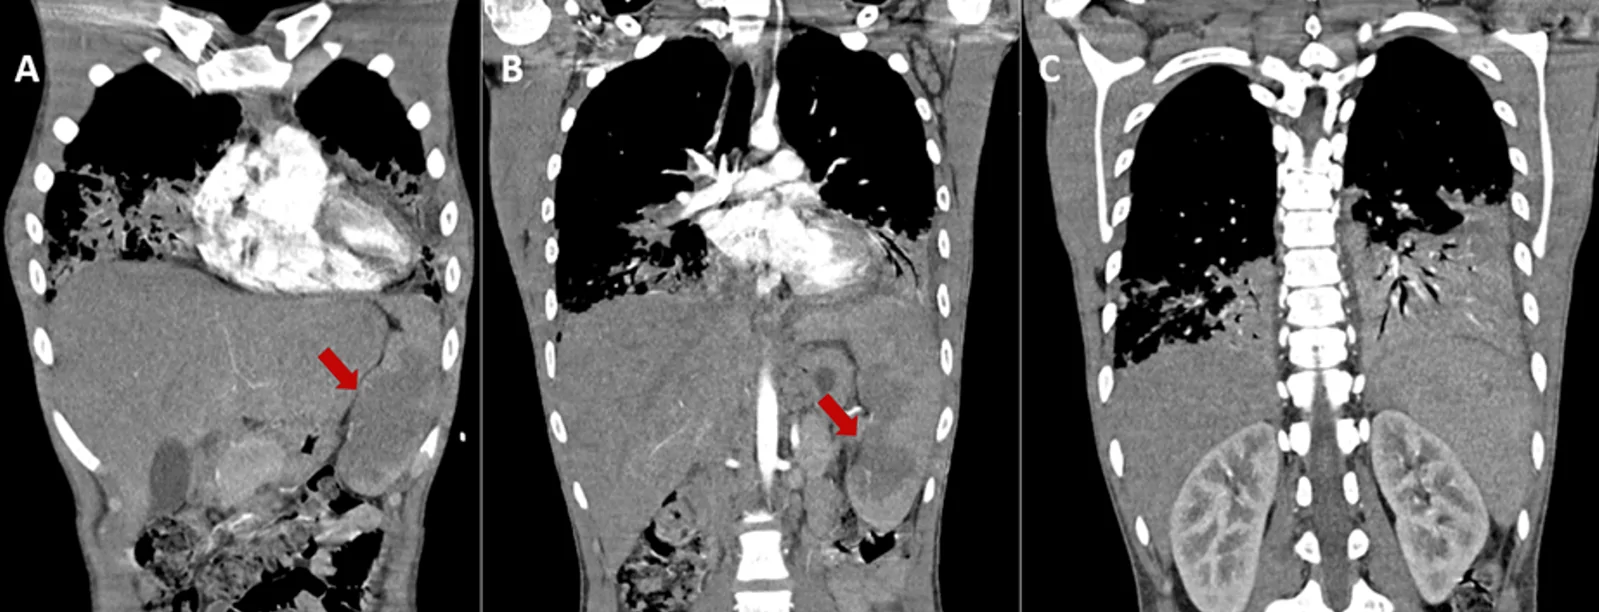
Coronal images of the contrast-enhanced chest, abdominal, and pelvic CT scan performed at the second hospital admission. Coronal images from the venous phase of the contrast-enhanced chest, abdominal, and pelvic CT scan obtained at the second hospital admission demonstrate bibasilar pulmonary consolidations with air bronchograms, predominantly involving the posterior segments of the lower lobes (image C). Hepatomegaly (image A) and splenomegaly (image B) are also identified. The spleen shows multiple hypodense lesions (red arrows), predominantly involving the perihilar and inferior aspects of the organ.
